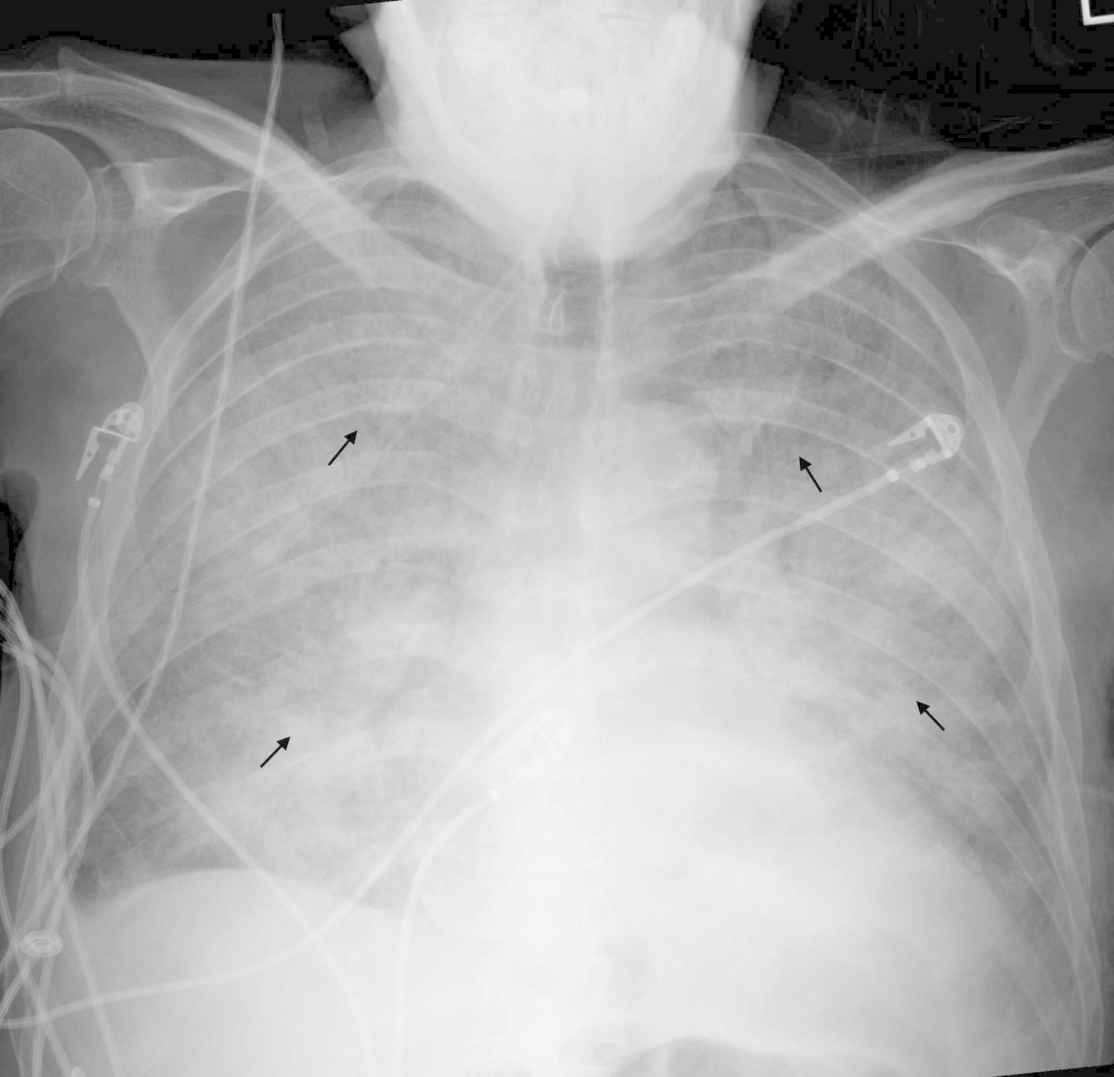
Portable anteroposterior chest radiography. Interval progression with development of extensive bilateral alveolar opacities (black arrows).
